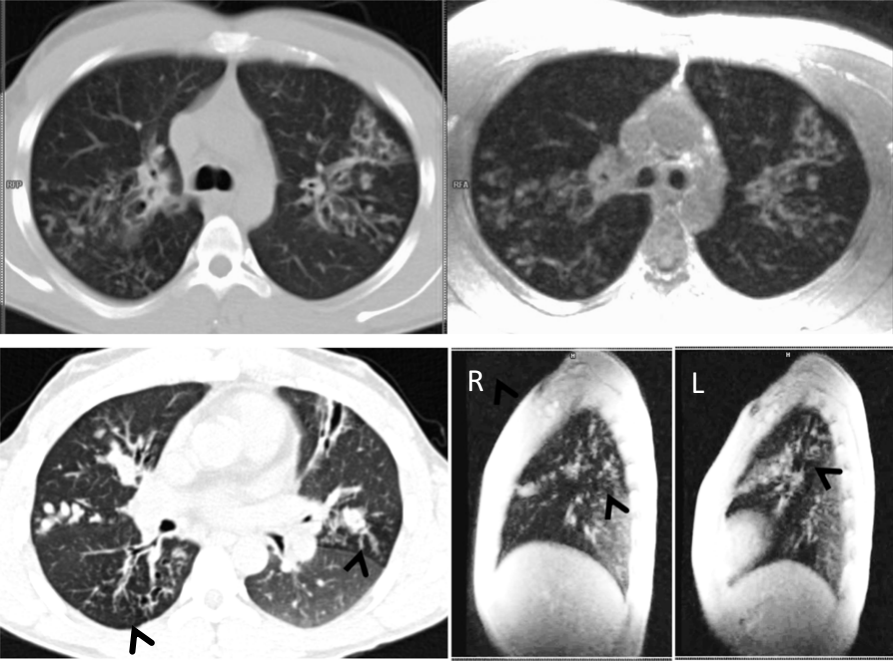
29 y/o male CF-patient, FEV1 predicted 67%. Left side CT, right side MRI at the same day. Upper row, transverse thin-section images from 3D acquisition in breath hold (CT and MRI). Note, despite lower resolution and signal to noise a similar depiction of bronchiectasis is possible. Lower row, expiration images (transverse CT and saggital MRI). In both modalities focal air trapping within the same lung region is demonstrated (arrow heads)
